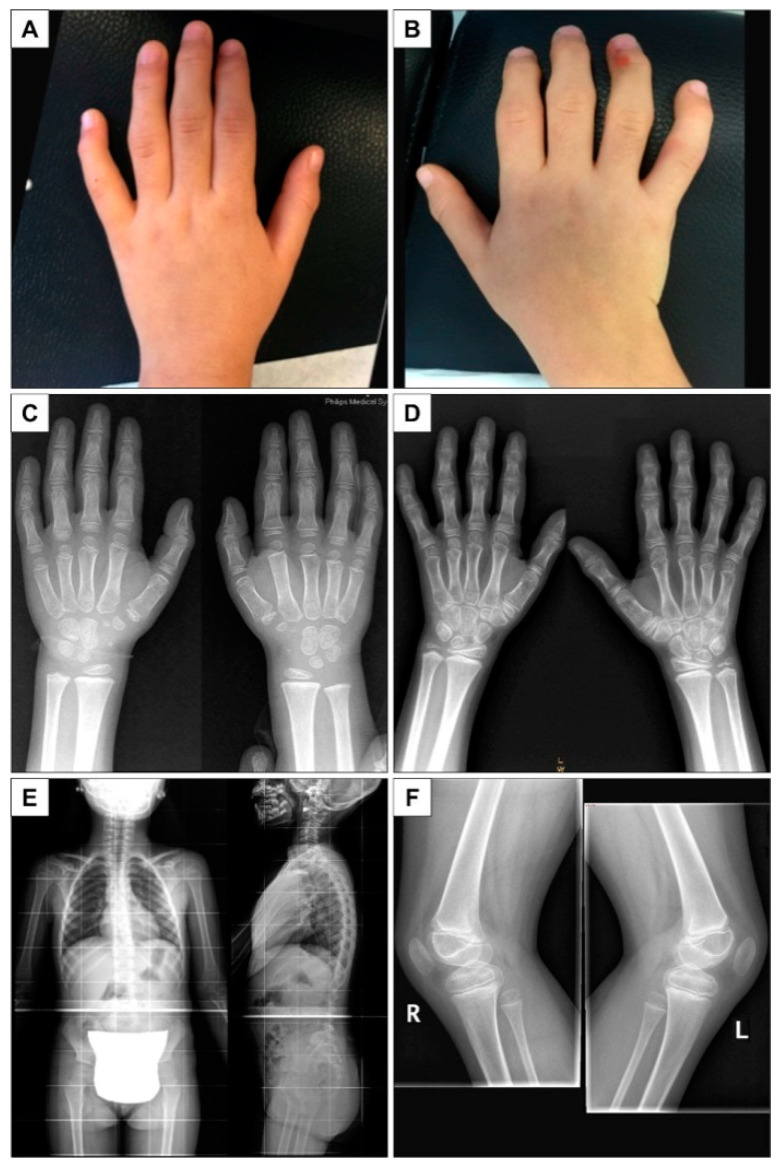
Clinical and X-ray images of the two siblings. Left: boy, right: girl. (A,B) First patient, age 6 years: swelling of the interphalangeal finger joints. (C) Second patient, age 4 years. Hand X-rays: enlargement and flattening of distal extremities of the proximal phalanges and enlargement and flattening of the proximal extremities of the middle phalanges, flat phalangeal epiphysis. Osteopenia. Bone age: carpus: 5 years, phalanges: 4 years 6 months (Greulick and Pyle method). (D) First patient, age 6 years. Hand X-rays: enlargement of proximal and distal phalangeal extremities, flat phalangeal epiphysis, crowded carpal bones. Osteopenia. Bone age: carpus: between 7 years 6 months and 8 years 6 months, phalanges: 5 years 6 months (Greulick and Pyle method). (E) First patient, age 6 years. Spine X-rays: slight platyspondily. Dorso-lumbar kyphoscoliosis. Bilateral coxa valga. (F) First patient, age 6 years 2 months. Knee X-rays: no double patella. Osteopenia.
